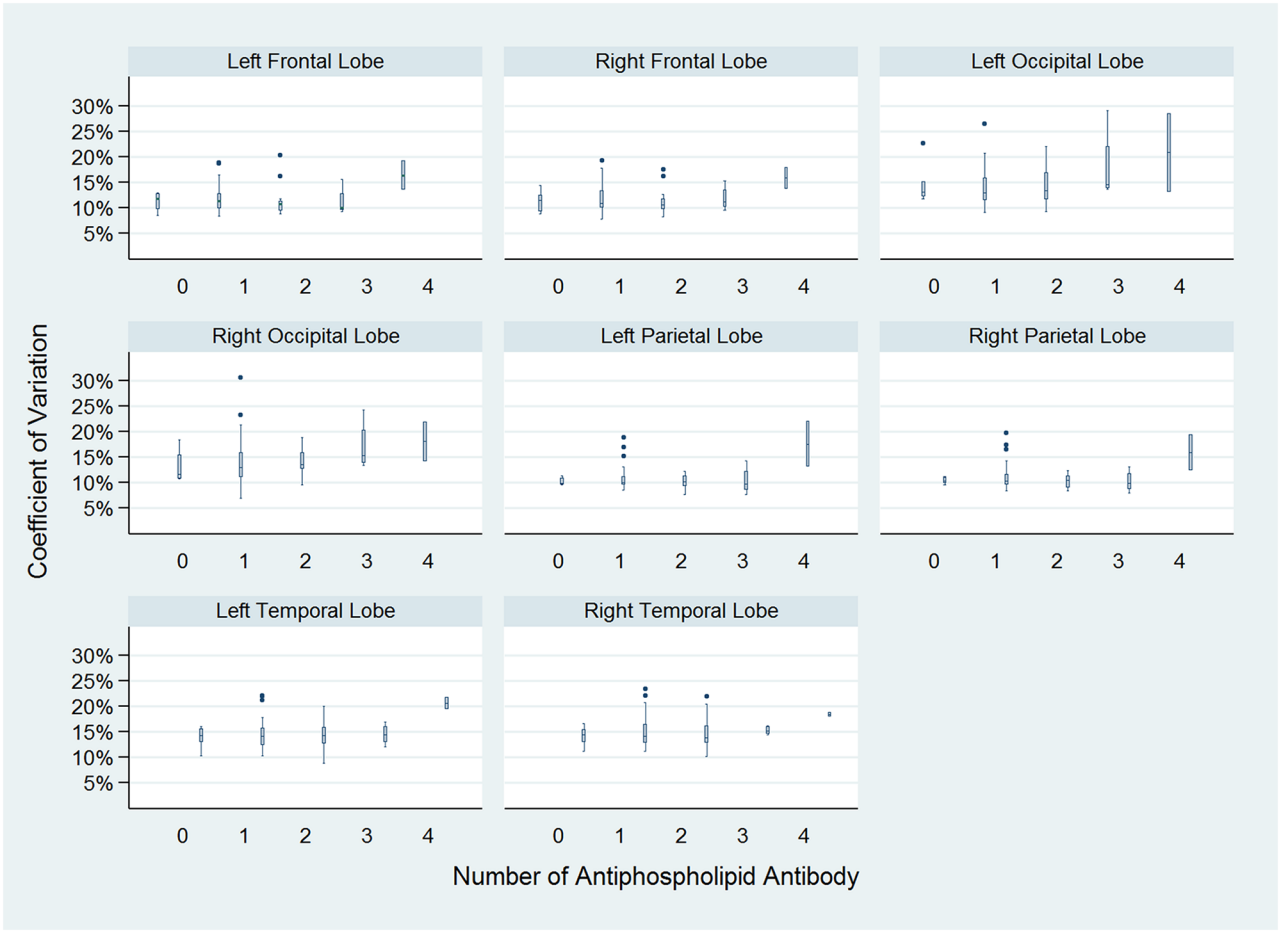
Heterogeneity of brain perfusion. The coefficient of variation of Tc-99m ECD SPECT signals is positively associated with the number of antiphospholipid antibodies (*P* = 0.01). The boxes represent interquartile range (IQR). The horizontal lines in the boxes represent median values; the horizontal lines out of the boxes represent 1.5 IQR. The black dots are outliers.
